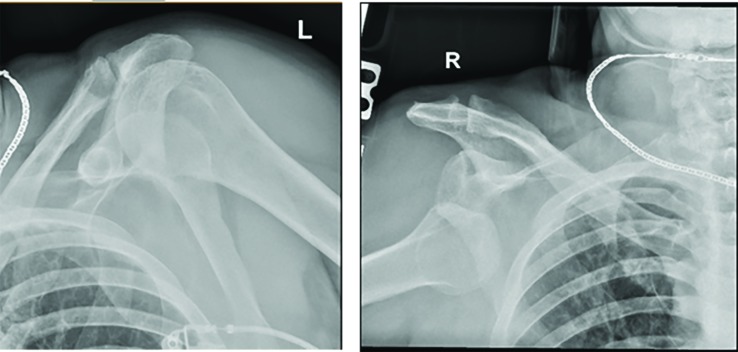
Bilateral shoulder radiography taken after initial attempted reduction, which demonstrates interval reduction of the left shoulder (Left) and right shoulder luxatio erecta (Right)
